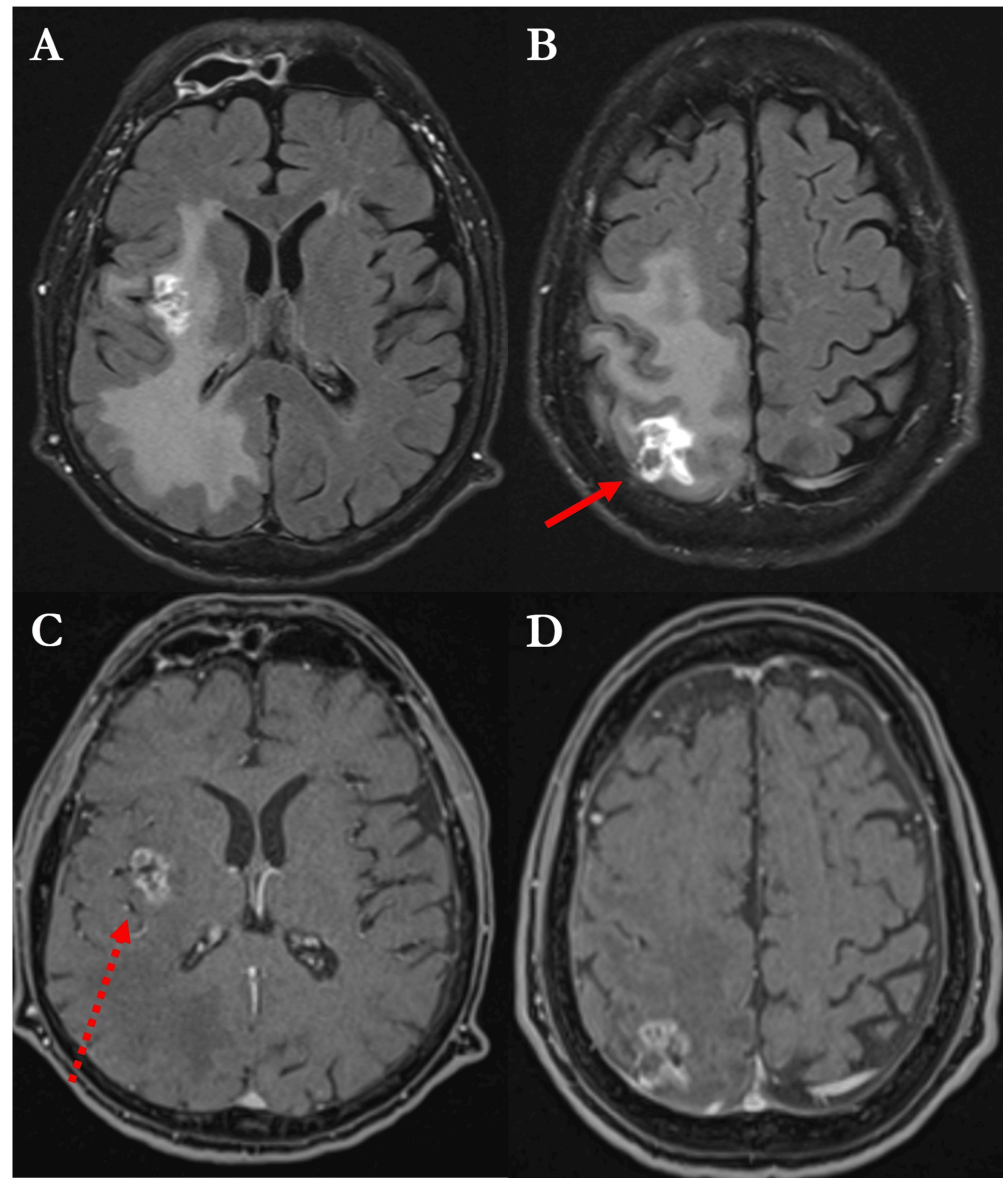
Preoperative contrast-enhanced brain MRI scan (A, B) Axial T2-weighted FLAIR sequences demonstrating a heterogeneously enhancing right parietal lesion characterized by central necrosis and surrounding vasogenic edema (solid arrow). (C, D) Axial T1-weighted contrast-enhanced images depicting the contrast-enhancing right parietal lesion.
